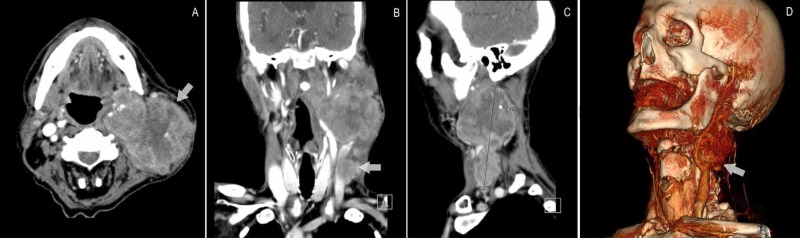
Cervical CT-scan on readmission, revealing incarceration of the left carotid sinus (A) Axial view; (B) coronal view; (C) sagittal view; (D) 3D reconstruction.
